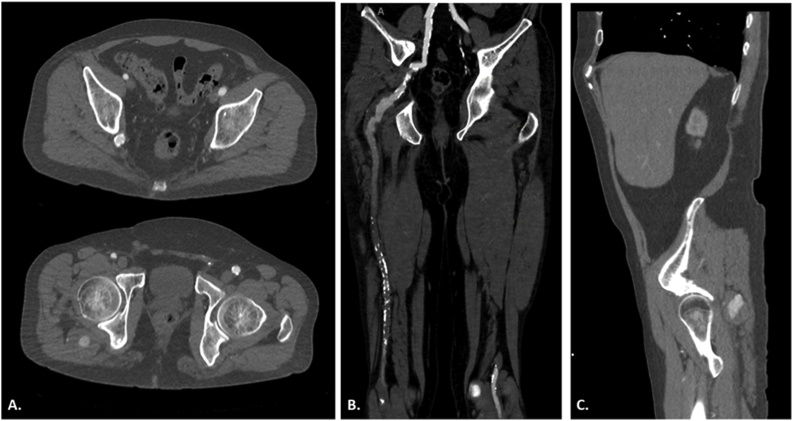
A) Computed tomography (CT) axial images at the gluteal region demonstrating a right-sided persistent sciatic artery (PSA) aneurysm. B) Coronal view of the posterior thigh elucidating the path of the right PSA from iliac artery to popliteal artery. C) Sagittal view confirming the size and location of the right PSA aneurysm.
